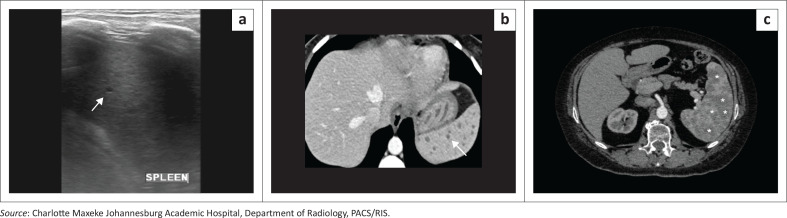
Granulomatous splenic disease in a patient with tuberculosis and in a separate patient with sarcoidosis. (a) Ultrasound and (b) axial contrast-enhanced CT images demonstrate multiple tiny hypodense splenic nodules in a patient with tuberculosis (arrows), consistent with splenic microabscesses. (c) Axial contrast-enhanced CT in a different patient demonstrates diffuse granulomatous infiltration of the spleen with innumerable well-circumscribed hypodense nodules (stars), consistent with sarcoidosis.
